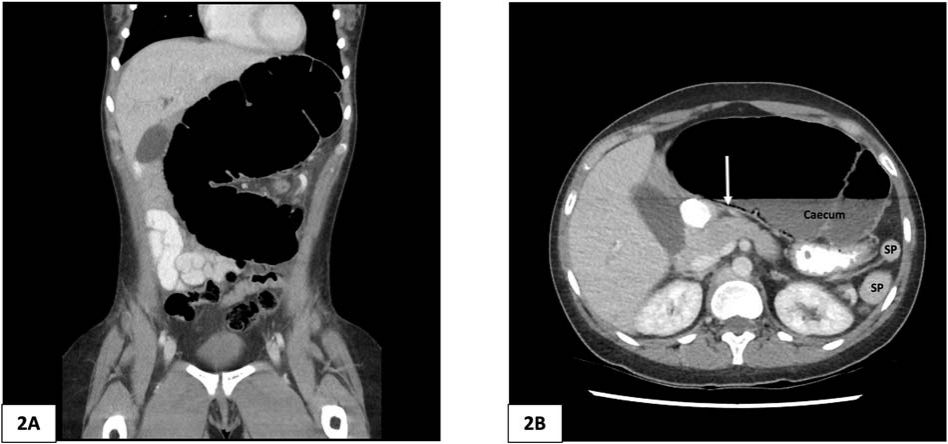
Computed tomography of the abdomen and pelvis of 25-year-old female patient. (A) Coronal slice demonstrating caecal volvulus with distended loop of colon. (B) Axial slice demonstrating 97 mm diameter distended caecum, multiple splenic fragments, arrow indicates pneumatosis coli, indicating colonic ischaemia. SP, spleen fragments.
